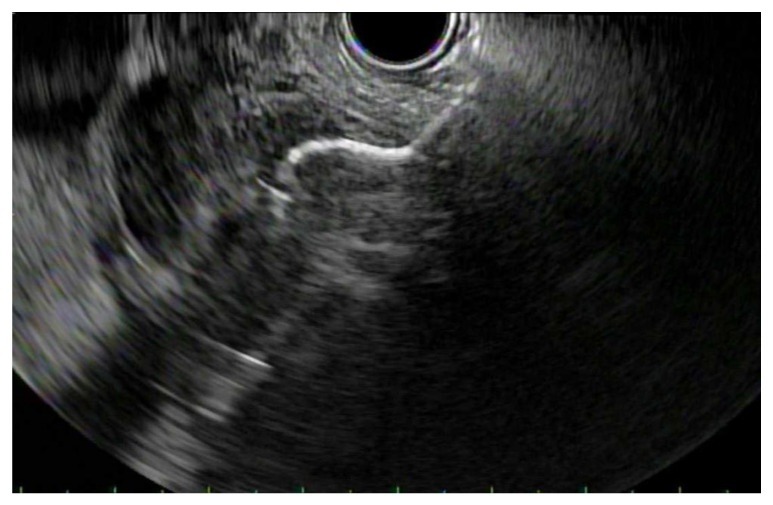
EUS-Gallbladder Drainage (EUS-GLB): Endosonographic image of a lumen-apposing metal stent being deployed between the gallbladder and the stomach.
